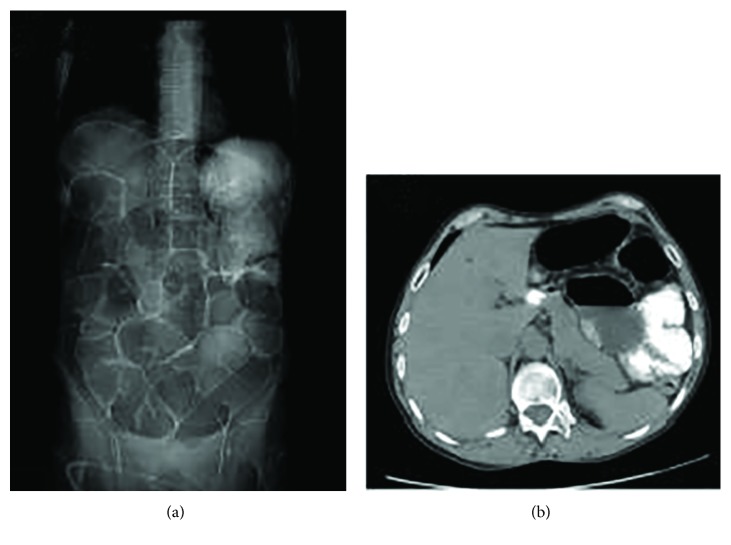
Radiological picture of BBS. Abdominal X-ray (a) and CT scan (b) show massive dilatation of the colon and the small intestine.
